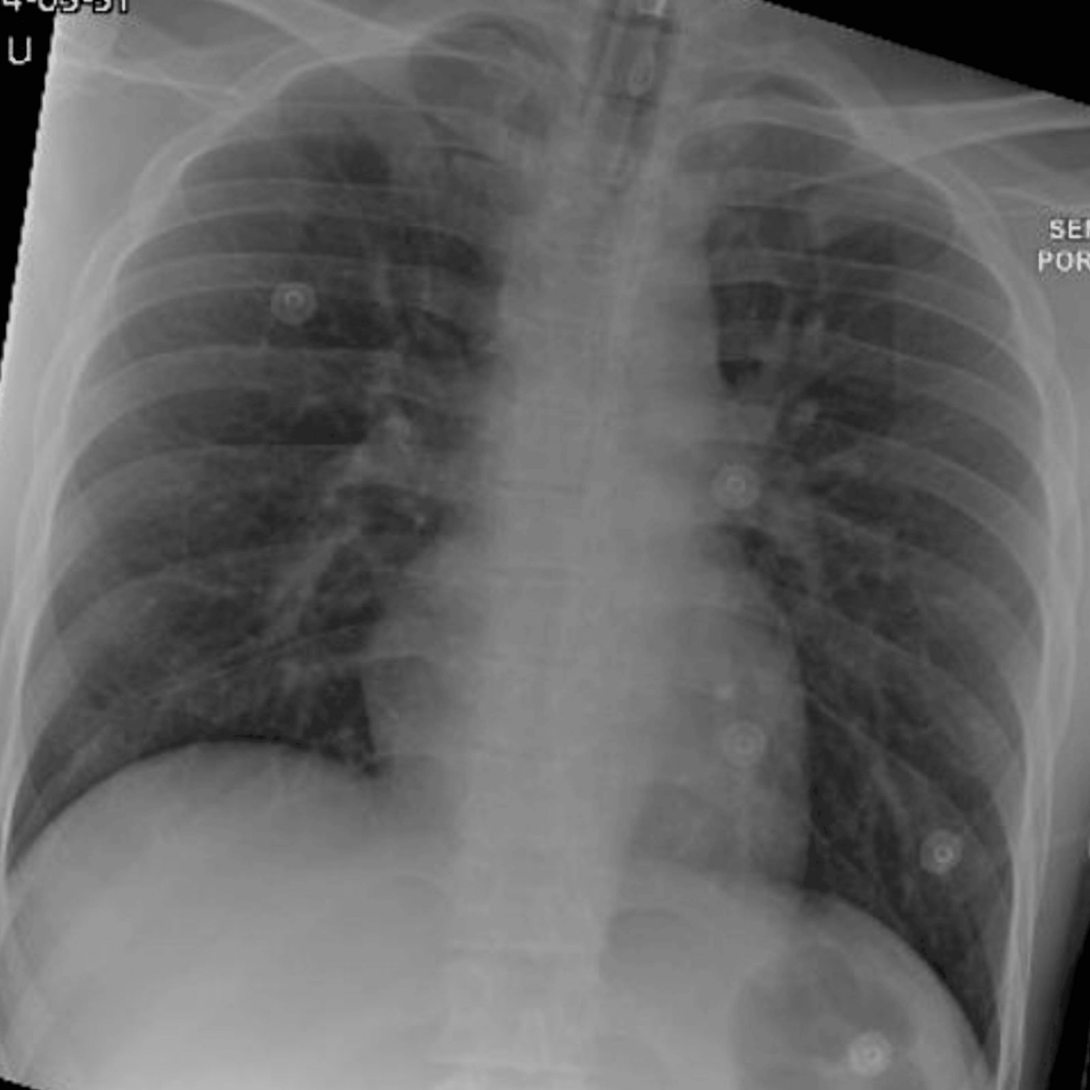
Chest X-ray, which shows an increase in the broncho vascular markings
